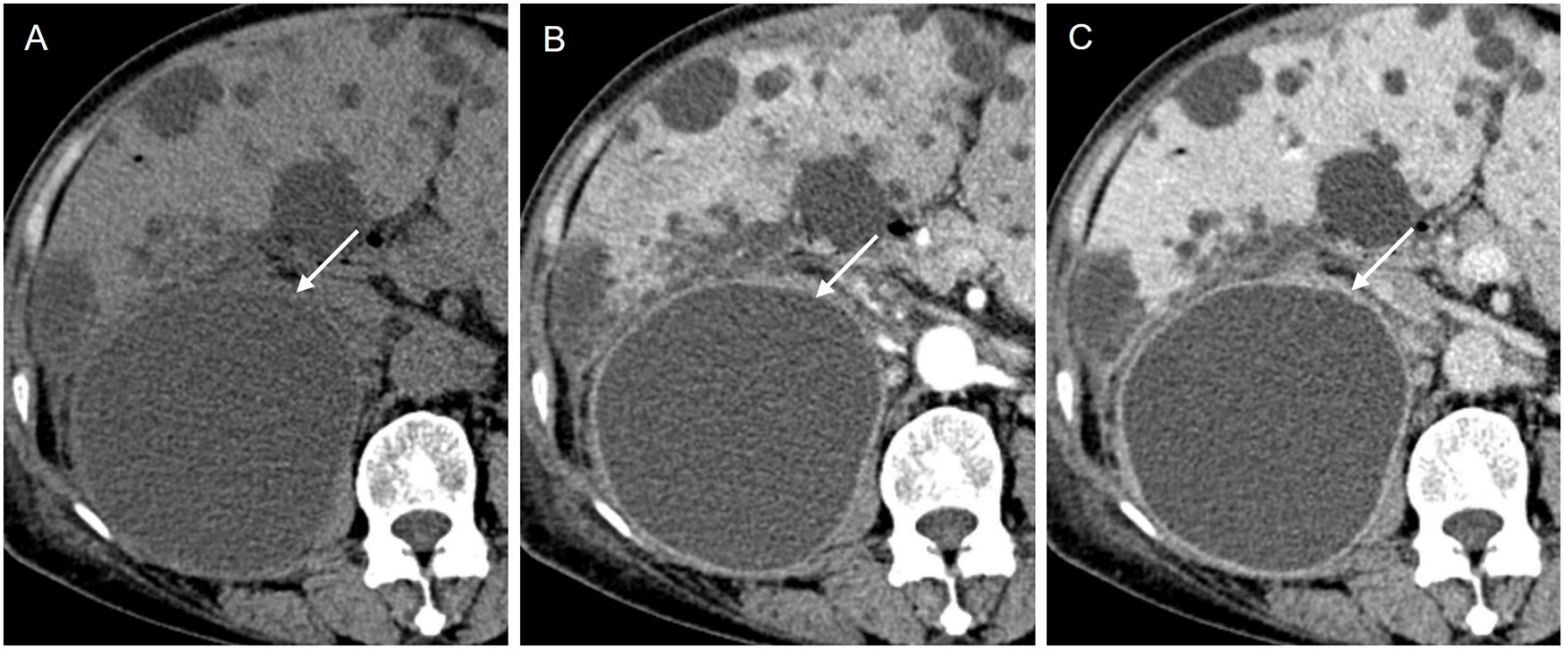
A 65-year-old female patient with an infected cyst (R24 in Fig 2). (A) Pre-contrast CT image shows a 9.7 cm sized round cyst that has higher attenuation than the surrounding cysts in right kidney upper pole (arrow). (B, C) On the arterial and portal venous phase images, the cyst shows discernible wall thickening with prominent wall enhancement and pericystic fat infiltration (arrows).
